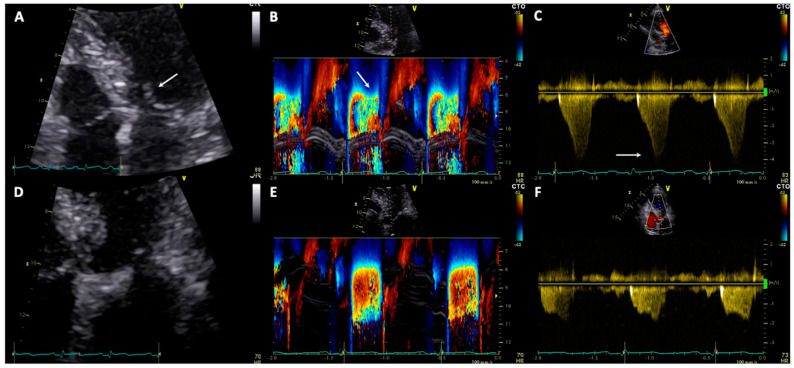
Panel (A) TTE with zoom on left ventricle outflow tract showing systolic anterior movement of anterior mitral leaflet during systole (see arrow); (B) Color M-Mode showing strong blood acceleration through left ventricle outflow tract in telesystole (see arrow); (C) CW-Doppler at TTE showing intraventricular peak gradient of 63 mmHg (see arrow); (D) TTE with zoom on left ventricle outflow tract showing absence of systolic anterior movement of anterior mitral leaflet during systole at recovery on day 6; (E) Color M-Mode showing regular blood acceleration through left ventricle outflow tract in telesystole at recovery; (F) CW-Doppler at TTE showing resolution of intraventricular gradient at recovery in the same patient. TTE: transthoracic echocardiography; CW: continuous wave.
